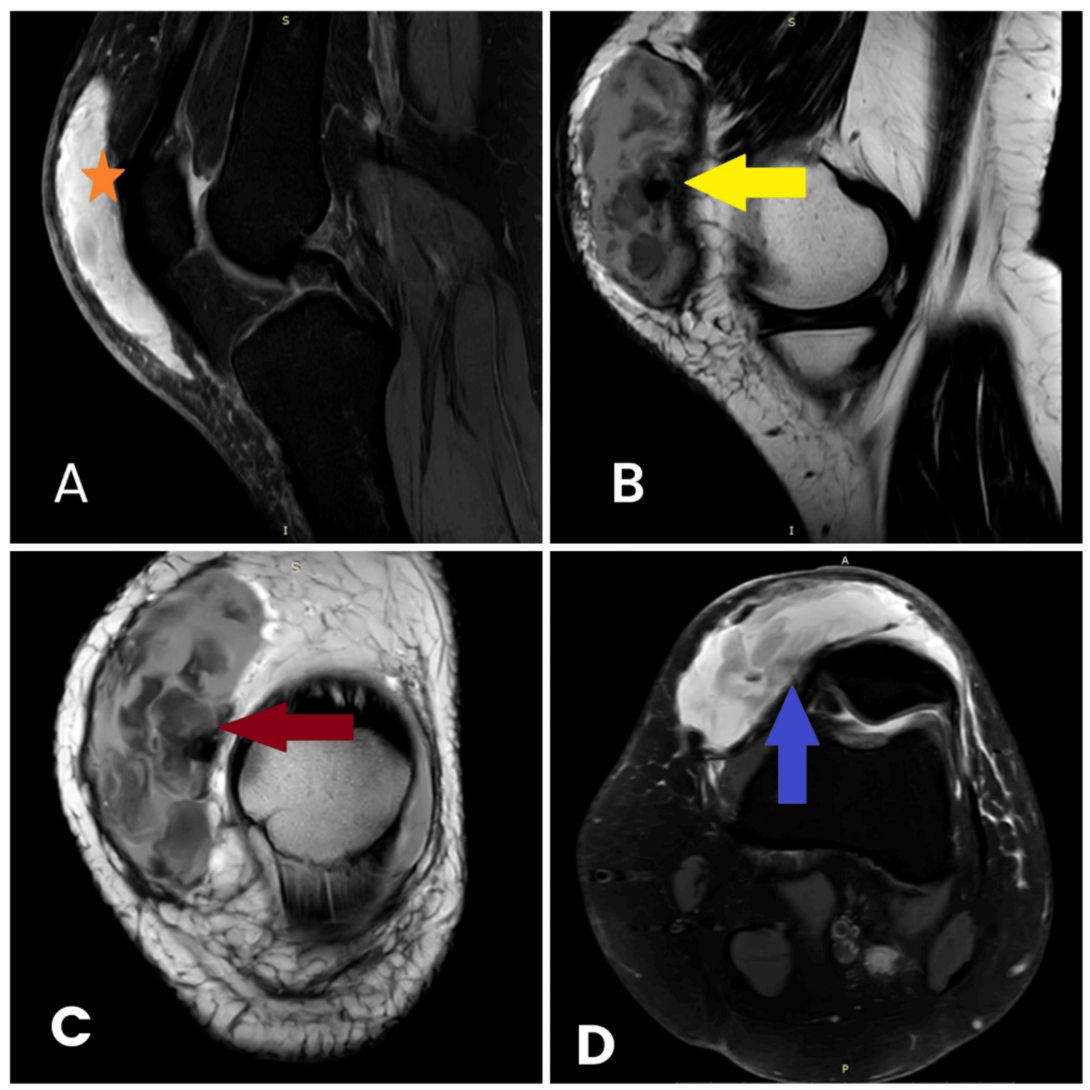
Preoperative MRI of the right knee. A: Sagittal section with prepatellar fluid collection (*). B: Sagittal section displays the extent of the prepatellar collection (yellow arrow). C: Coronal section with the red arrow pointing to the collection. D: Transverse section with the blue arrow pointing to the collection.
